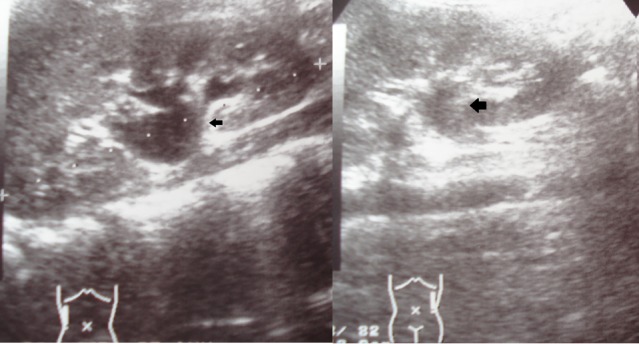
Ultrasound of the abdomen showing bilateral hydronephrosis (right more than left) as pointed by the black arrows.
